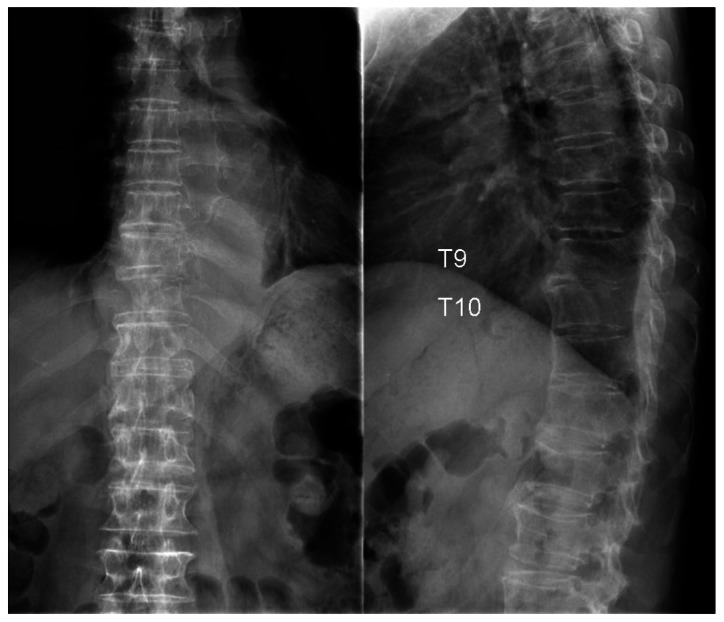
The AP and lateral X-ray showed lytic involvement of T9 and T10 vertebral pedicles and posterior wall.
